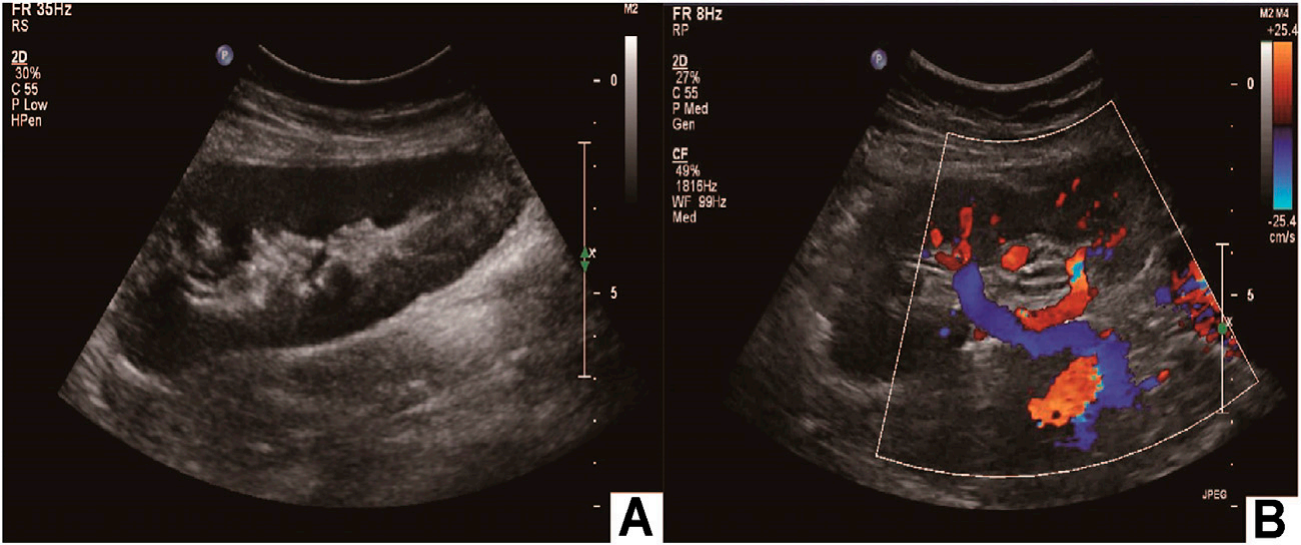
Postoperative imaging confirmed autotransplantation success. (A) Doppler ultrasound of autotransplant showing that the transplanted kidney was of normal size and shape; (B) the transplanted kidney was supplied by the right external iliac artery, and the vein returned to the right external iliac vein.
